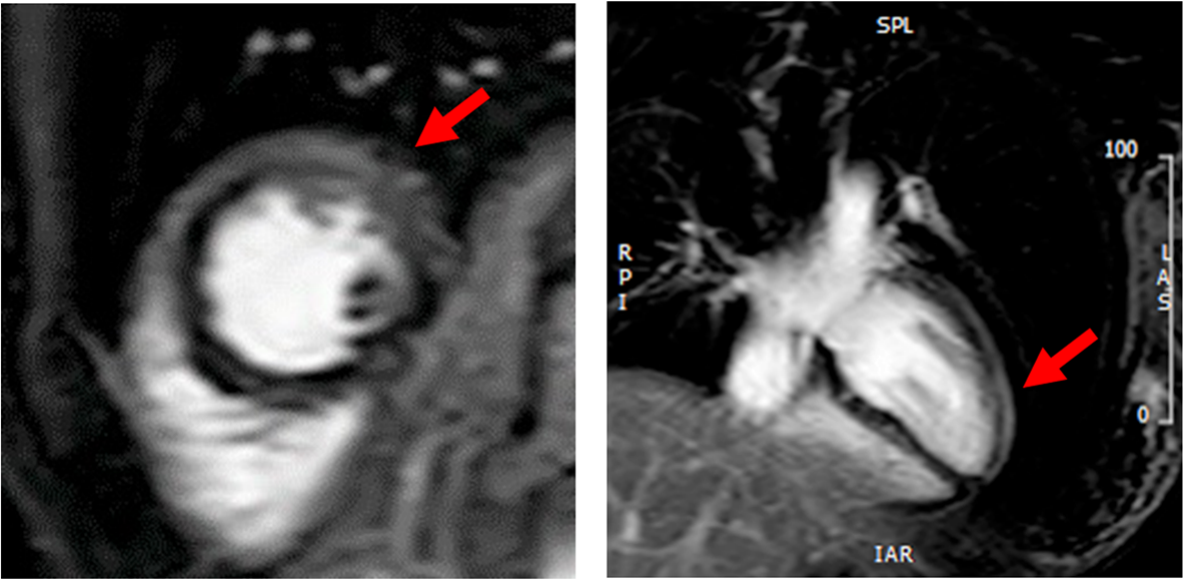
Cardiac MRI. LGE was observed in the apical wall (indicated by filled arrow). LGE: late gadolinium enhancement
